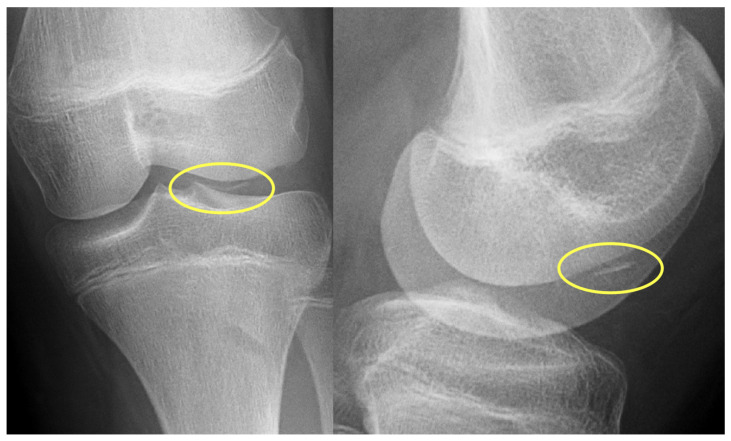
Preoperative X-ray showing the osteochondral fragment (yellow circle) of the lateral condyle of the femur.
